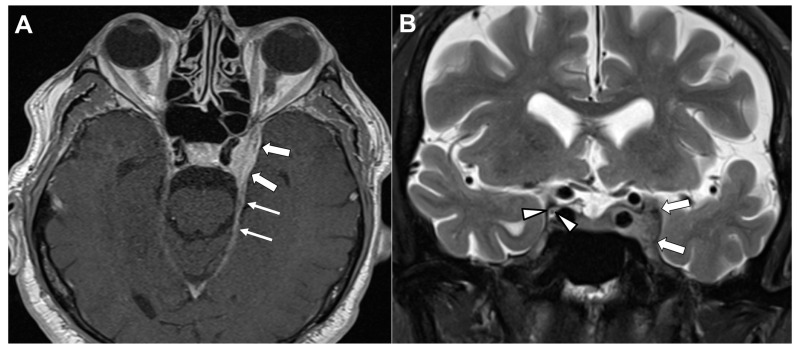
MRI features of left cavernous sinus meningioma with oculomotor nerve (OMN) involvement. (A) Axial T1-weighted post-contrast (non-fat-saturated) image demonstrates a homogeneously enhancing soft tissue lesion filling and expanding the left cavernous sinus (thick arrows), encasing the cavernous segment of the internal carotid artery without significant luminal narrowing. Contrast enhancement extends posteriorly along the tentorium (thin arrows), a finding known as the “dural tail sign”, supporting a dural-based neoplasm. (B) Coronal T2-weighted fat-saturated image shows the lesion to be iso- to mildly hypointense relative to gray matter, with mass effect upon the lateral wall of the cavernous sinus and obscuration of the expected course of the OMN. Note how the right OMN is clearly visualized within the right normal cavernous sinus (arrowheads), whereas the left OMN cannot be distinguished due to its compression (arrows). The imaging features are characteristic of a cavernous sinus meningioma. The patient had presented with progressive ptosis, diplopia, and impaired extraocular movements. Image Credit: Dr. Nikolaos-Achilleas Arkoudis and Prof. Georgios Velonakis.
